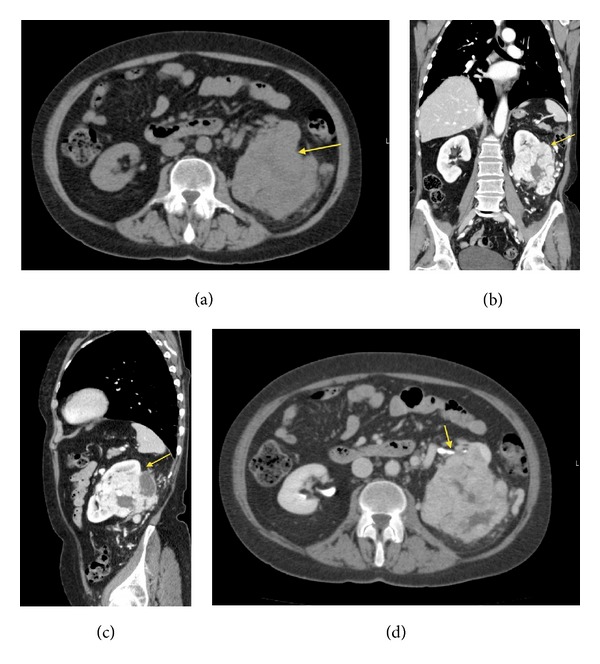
(a) and (b) show the presence of the renal lesion with multilobulated shapes, in the left kidney, 90 mm (LD) × 85 mm (TD) × 96 mm (APD) in size. (c) and (d) show the extension and the location of the renal lesion, mass infiltrates the renal pelvis and the mid calyx (c), and proximal ureter was encased but not infiltrated (d). Arrows indicate the lesion. (a) Axial CT scan, precontrast phase. (b) CECT, multiplanar reconstruction (MPR) Coronal Plane. (c) CECT-MPR: Sagittal Plane. (d) Axial-CECT, urographic phase.
